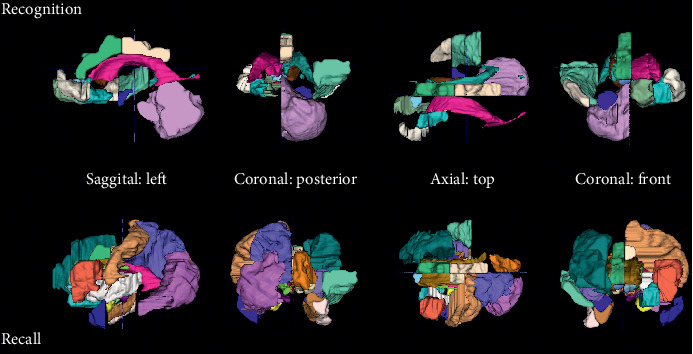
MRI regions selected for prediction of executive functions decline. For the combination with EEG during recognition and neuropsychology, volumetry from the following regions was selected as features: amygdala right, caudate nucleus right, medial, lateral, and posterior orbital gyrus left, superior temporal gyrus middle part right, bilateral lateral ventricle excluding temporal horn, subcallosal area left, bilateral pre-subgenual frontal cortex left, cerebellum right, superior temporal gyrus anterior part right, and anterior and posterior cingulate gyrus right. For the combination with EEG during recall without neuropsychology, volumetry from the following regions was selected as features: bilateral amygdala, anterior temporal lobe lateral part left, parahippocampal and ambient gyrus right, superior temporal gyrus middle part right, fusiform gyrus right, bilateral insula, lateral remainder occipital lobe left, anterior cingulate gyrus right, bilateral posterior cingulate gyrus, middle frontal gyrus right, bilateral caudate nucleus, bilateral nucleus accumbens, thalamus right, corpus callosum, lateral ventricle excluding temporal horn left, inferior frontal gyrus left, postcentral gyrus left, superior parietal gyrus left, cuneus right, posterior orbital gyrus left, substantia nigra left, subgenual frontal cortex left, subcallosal area right, and bilateral pre-subgenual frontal cortex L.
